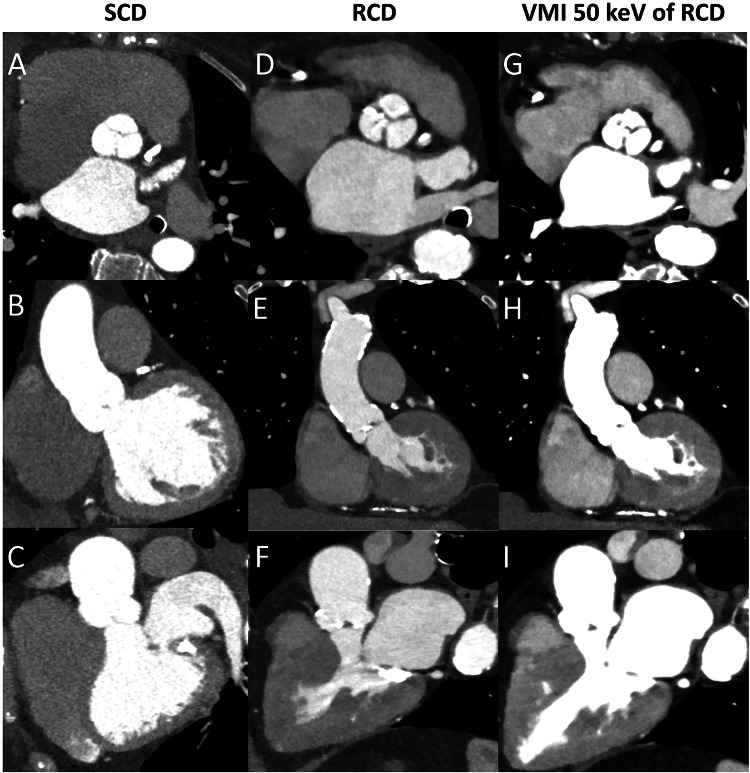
Representative axial, coronal and sagittal images (window width/window level: 700/80 HU) of a patient acquired with standard contrast media dose (SCD) (A, B, and C), a BMI-matched patient with reduced contrast media dose (RCD) (D, E, and F), and corresponding virtual monoenergetic images (VMI) of RCD at 50 keV (G, H, and I) acquired with a photon-counting detector CT (PCDCT)
